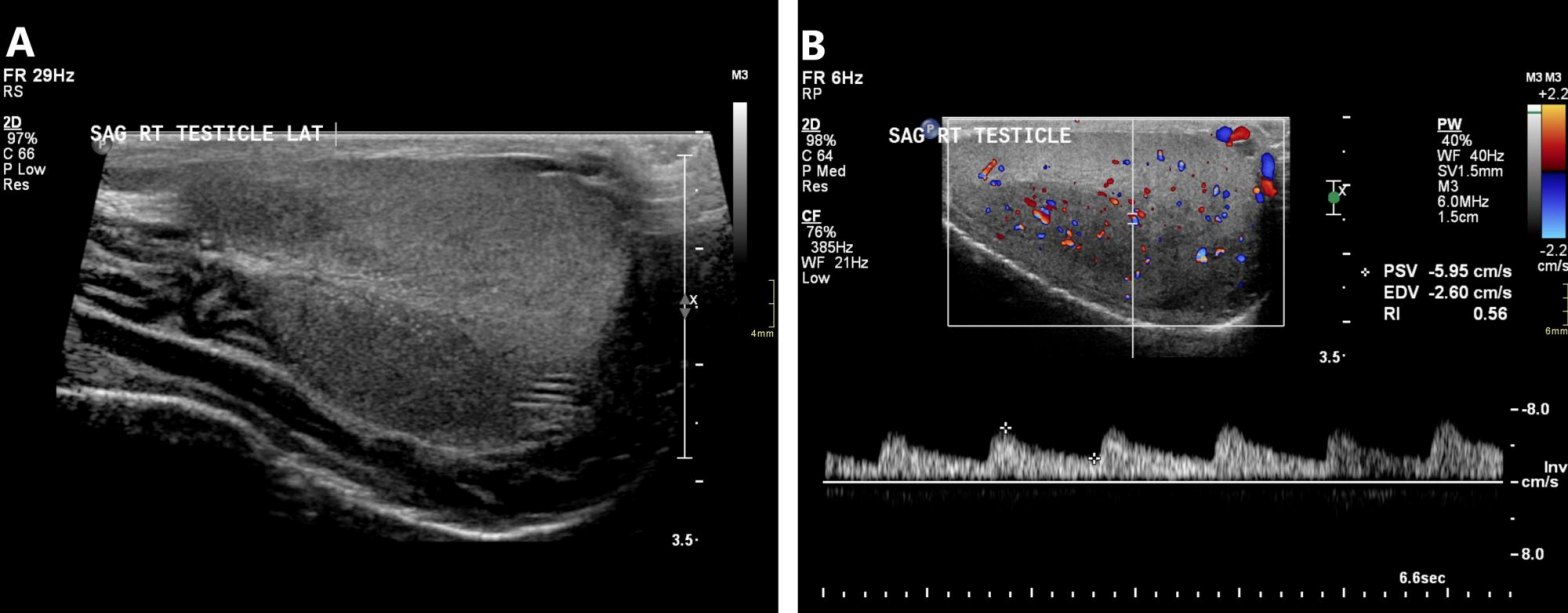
Right testicular lymphoma. (A) Within the right testis, there is a suspicious hypoechoic region posterior to the mediastinum testis. (B) Doppler interrogation reveals mild hyperemia in this hypoechoic region.
